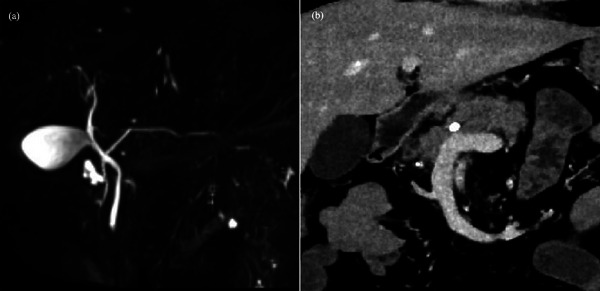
(a) Magnetic resonance cholangiopancreatography showing pancreas divisum. (b) Contrast‐enhanced computed tomography shows a pancreatic stone; the main pancreatic duct does not dilate.
